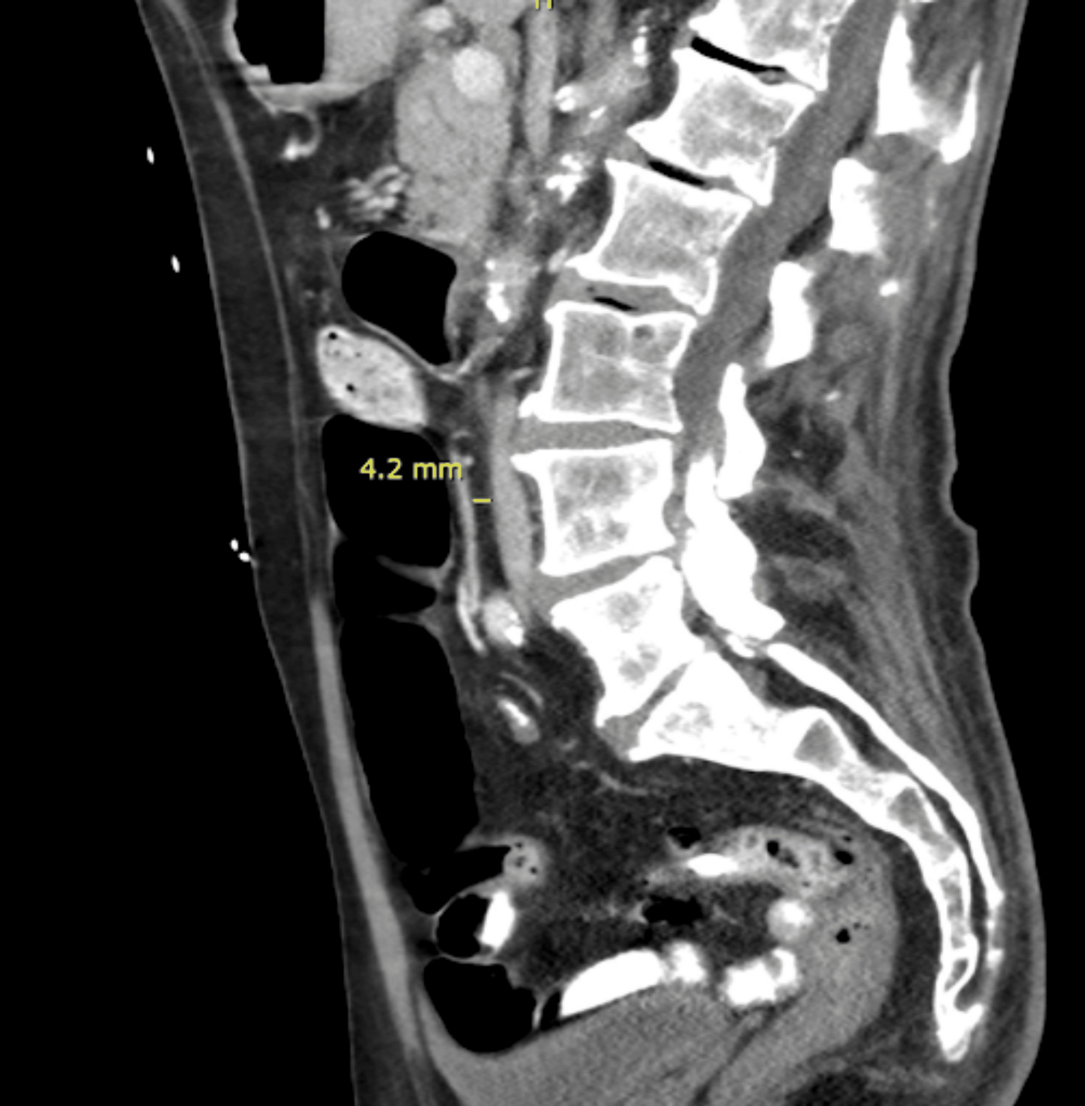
Sagittal contrast-enhanced CT image of the abdomen. Contrast-enhanced sagittal CT image of the abdomen demonstrating compression of the third portion of the duodenum between the SMA and the aorta. The measured aortomesenteric distance is 4.2 mm (yellow line).
